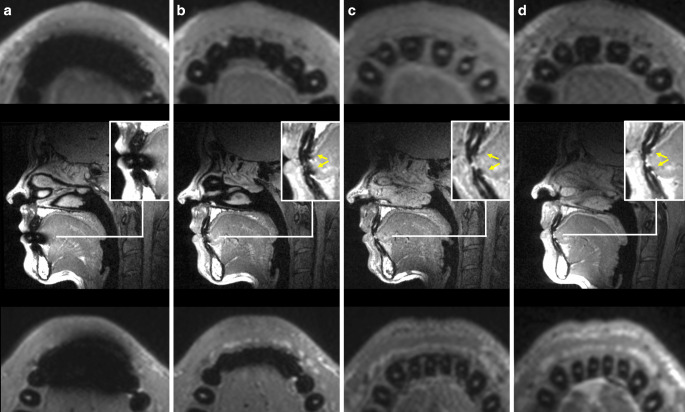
MRI images: Transversal plane of upper jaw (*top*), sagittal plane (*middle*), transversal plane of lower jaw (*bottom*); artefacts for CoCr (b), NiTi (c) and Ti5 (d) retainers marked with *yellow arrows*—twistflex retainers caused artefacts which impaired the visibility of both non-dental and dental landmarks (a). CoCr retainers impaired the visibility of the pulp and the incisal edges (b). NiTi (c) and Ti5 retainers (d) had no impact on the visibility of both non-dental and dental landmarks. *CoCr* cobalt–chromium, *NiTi* nickel–titanium, *Ti5* grade‑5 titanium MRT-Aufnahmen: Transversale Ebene des Oberkiefers (*oben*), sagittale Ebene (*Mitte*), transversale Ebene des Unterkiefers (*unten*); Artefakte von CoCr- (b), NiTi- (c), Ti5- (d) Retainern mit *gelben Pfeilen* markiert – Twistflex-Retainer verursachten als einzige Retainer Artefakte, welche die Sichtbarkeit von dentalen und nichtdentalen Landmarken beeinträchtigten (a). CoCr-Retainer beeinträchtigten die Sichtbarkeit der Pulpae und Inzisalkanten (b). NiTi- (c) und Ti5-Retainer (d) hatten keinen Einfluss auf die Sichtbarkeit dentaler und nichtdentaler Landmarken. *CoCr* Cobalt-Chrom, *NiTi* Nickel-Titan, *Ti5* Titan Grad 5
